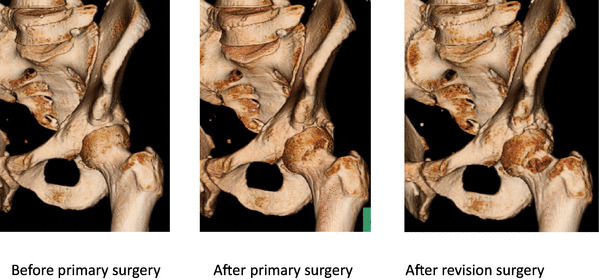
Images of 3D-CT before the primary operation, postoperatively, after revision surgery. It shows that the bulge of AIIS remaining after primary operation has disappeared after revision surgery
